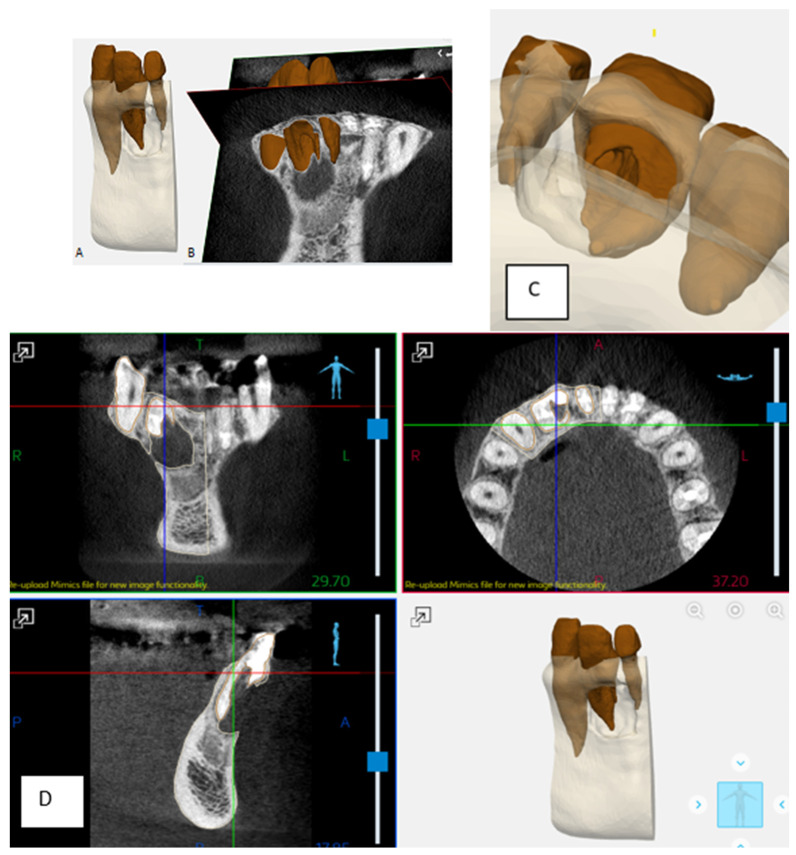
Renderings used during the design process. (A,C) Teeth #25–#27 within the alveolus. Both the DI and the lesion are modeled. (B) Teeth #25–#27 with overlaid axial and coronal CBCT sections. (D) CBCT imaging used to design 3D tooth model with mapping of the DI and surrounding tissues.
